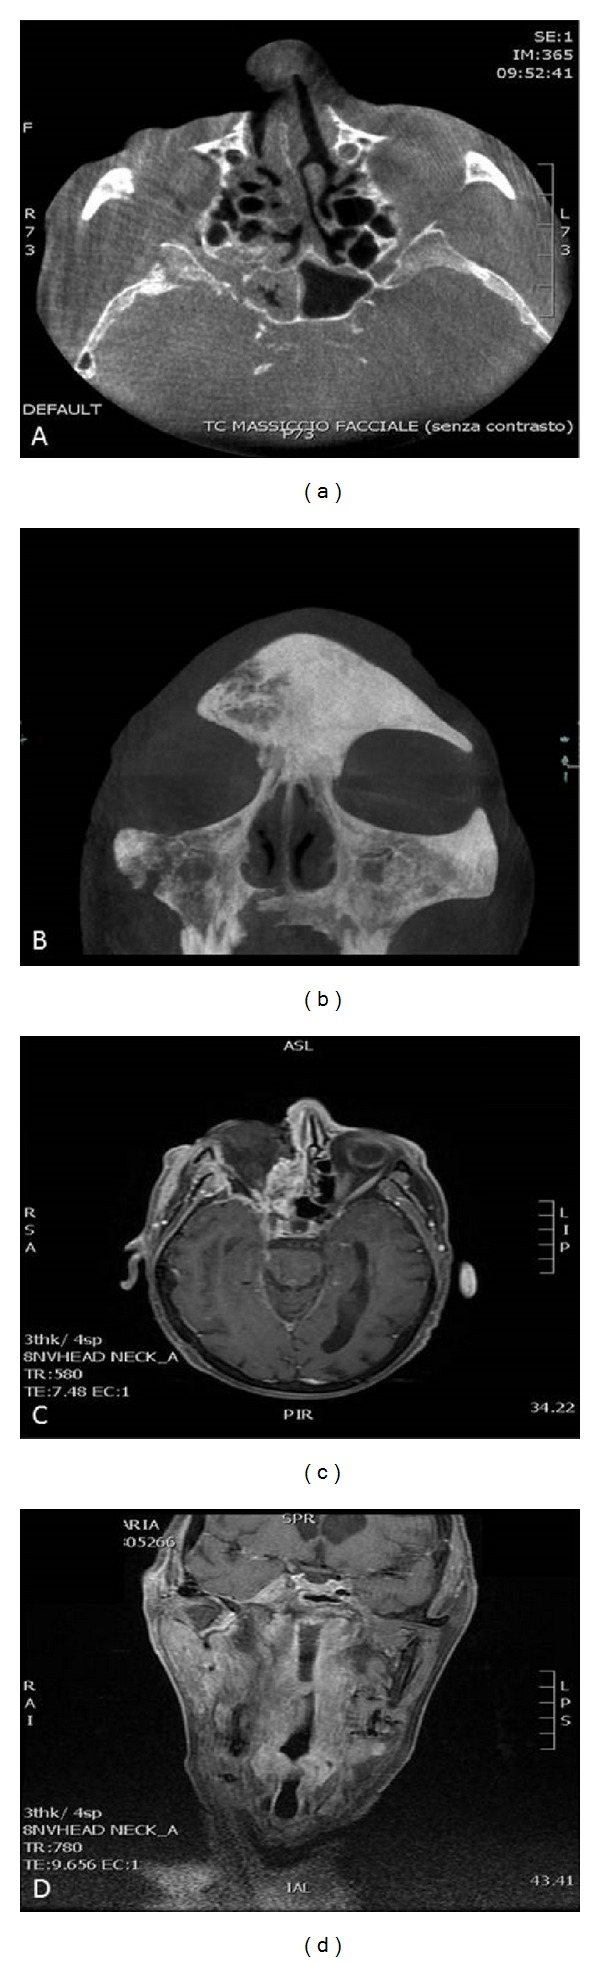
Base (axial plane) CT image shows the presence of a soft tissue density mass in the right sphenoid sinus, occluding the sinus lumen and the spheno-ethmoid osteum also osteostructural alterations, mainly lytic, of the lateral sinus wall and in part of the large sphenoid wing (a); base (coronal plane) CT image with MIP (maximum intensity project) reconstruction shows lytic osteostructural alteration of the right side of the hard palate, with interruption of the cortical contour and, in part, of the alveolar process of the jaw in the first sextant (b); axial T1-weighted MRI image after mdc administration shows meningeal enhancement in the right temporal lobe. Also enhancement of the temporiun on the right side and the Sylvian aqueduct indicating transcompartment fungal infection involvement (c); coronal T1-weighted MRI image after administration of mdc shows a large amount of phlogistic tissue, intensely and homogenously enhanced after mdc, which fills the Meckel's cavity and engulfs the intracavernous branches of the internal carotid artery in the absence of perceptible ischaemic lesions of the brain parenchyma (d).
